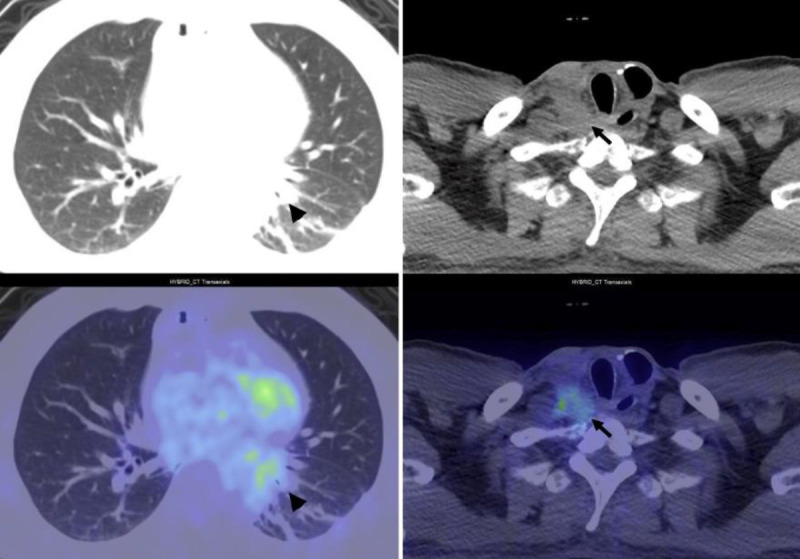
^18^F-FDG PET/CT fusion images demonstrate two distinct lesions: (A) A mildly ^18^F-FDG-avid lesion in the left lower lobe of the lung, infiltrating the left lower lobar bronchus with associated bronchial narrowing (arrowheads). (B) A hypermetabolic soft-tissue lesion in the right cervical region, adjacent to the upper trachea at the C7–T1 level, near the esophagogastric anastomosis (arrows)
